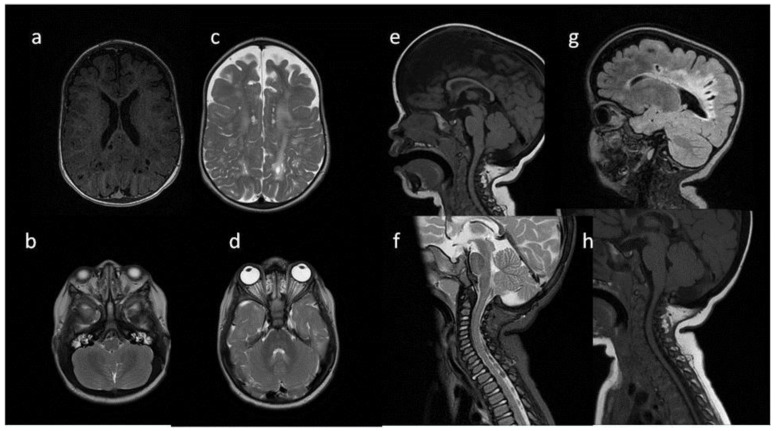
Brain and cervical spine MRI. Patient 4, follow up: (a,b,h) axial T2; (c,d,g); sagittal brain (c) T1; (d,g) T2; (e,f) sagittal spine (e) T2, (f) T1. Findings: (a–d) enlarged PVS with characteristic location in corpus callosum; (a,b,d,g) abnormal white matter signals;(e,f) vertebral spaces and bodies deformity, J-shaped sella turcica, dysplastic dens, (h) mastoid cells fluid, discrete posterior fossa horns, (a–d,g) skull deformity and enlarged frontal subarachnoid spaces.
